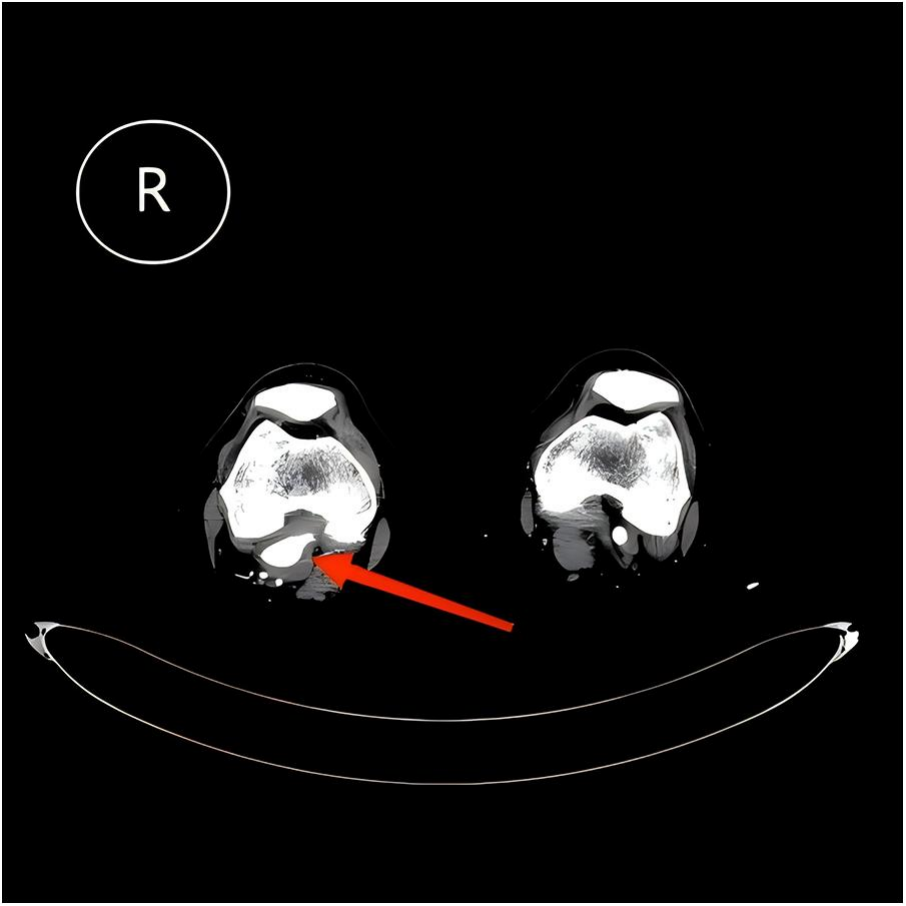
Ultrasound image showing a right popliteal artery aneurysm (4.1 cm) with a 15 mm mural thrombus; the residual lumen measures 2.2 cm. Arrow indicates the popliteal artery aneurysm.
